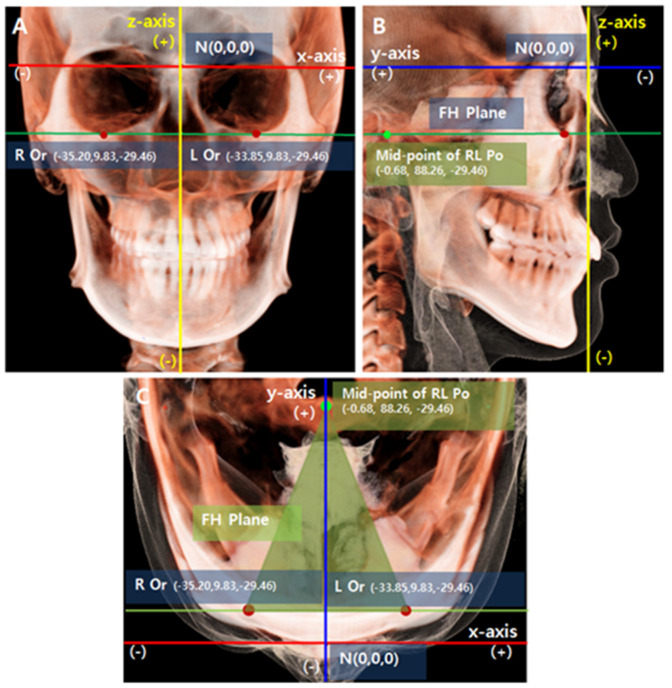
The coordinate system and standard reorientation of the CBCT Images. (A) Coronal view. (B) Sagittal view. (C) Axial view. The coordinate system consists of three axes (x, y, z) with their origin (0,0,0) registered at nasion (N). The Frankfort horizontal plane (FH plane) was constructed with bilateral orbitale (Or) points and the midpoint of bilateral porion (Po) points. The orbit line is a line passing both orbitale points in 3D space. The x-axis (red line) is a transverse axis passing through the N point and is also parallel to the FH plane in the coronal plane. The y-axis (blue line) is the anteroposterior axis passing through the N point and perpendicular to the x-axis. The y-axis is parallel to the FH plane in the sagittal plane. The z-axis (yellow line) is the vertical axis perpendicular to both the x- and y-axes. The x-axis is also parallel to the orbit line in the axial plane. Positive values are to the left, posterior, and superior (LPS) of the N point of the subject. Negative values are to the right, anterior, and inferior (RAI) of the N point.
